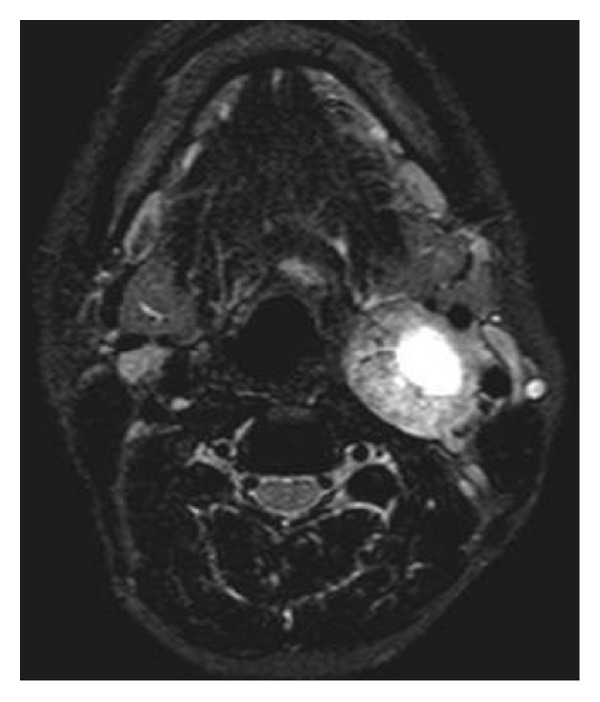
T2-weighted MR image shows hyperintense mass with central cystic component.
